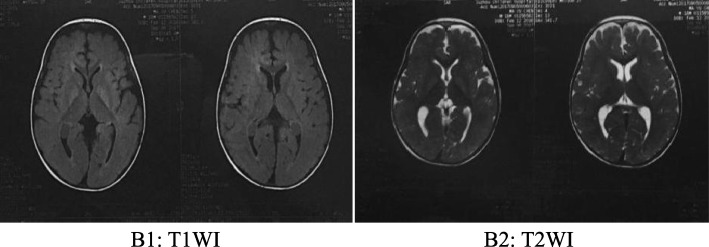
The MRI at the age of 2.5 years: The bilateral cerebral hemisphere of gray matter and white matter is well demarcated, and the symmetrical long T2 and DWI of central gyrus, corona radiata, posterior limbs of internal capsules, thalamic region and cerebellar dentate nucleus have improved than before.The abnormal signal of inner capsule basically disappeared.
